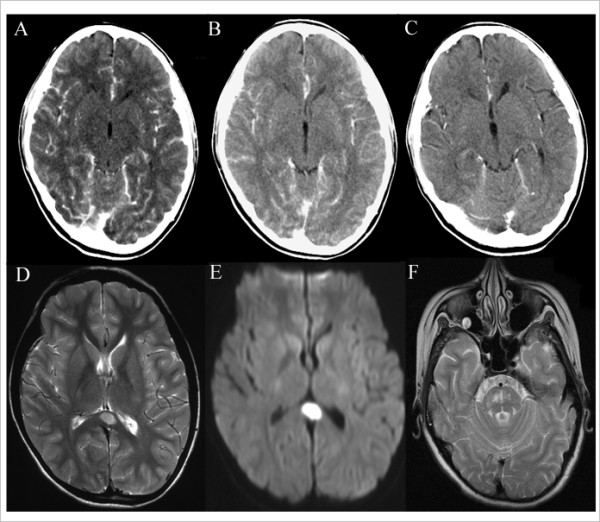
Cranial computed tomography scans obtained before normothermic treatment and during follow-up. A cranial computed tomography scan obtained on day 5 (A) before normothermic treatment, showing remarkable meningeal enhancement and brain swelling. Follow-up computed tomography scans obtained on day 12 (B) and day 29 (C), showing reduced meningeal enhancement and brain swelling after normothermic treatment. T2-weighted magnetic resonance imaging (D) and diffusion-weighted magnetic resonance imaging (E) scans, showing increased signal intensity of an ovoid lesion in the splenium of the corpus callosum. A T2-weighted magnetic resonance imaging scan obtained on day 48, showing abnormal increased signals in the pontine (F).
